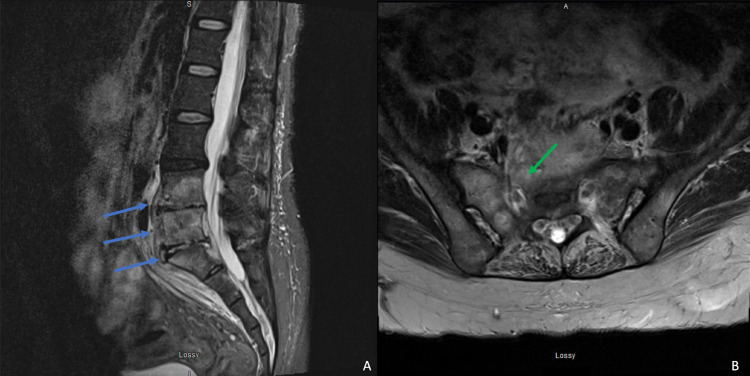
MRI at four months. Sagittal STIR (A) and axial T2 (B) images revealing stabilization of lesions in L4-S1. MRI: magnetic resonance imaging; STIR: short tau inversion recovery
